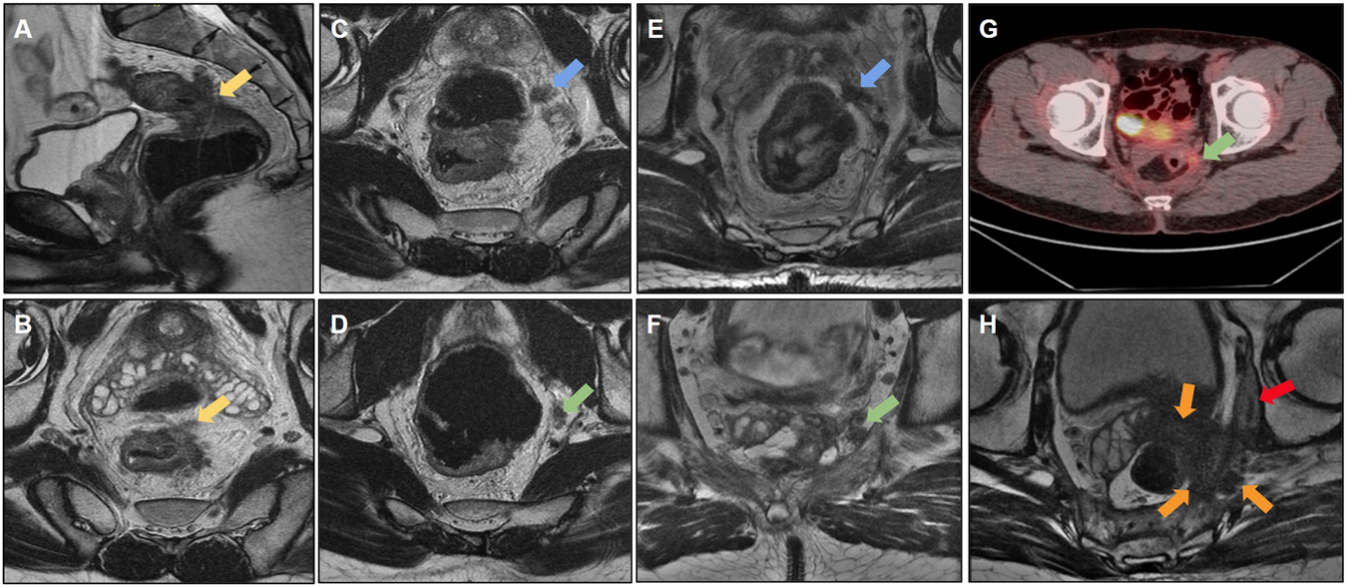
A, B Sagittal and axial T2-weighted image: baseline mrT4aN1c (yellow arrow), mesorectal fascia (MRF) positive and extramural vascular invasion positive (EMVI). C Tumor deposit in the rectoprostatic recess (blue arrow) and (D) suspicious lateral pelvic lymph node on the left (green arrow) were also characterized. E Post-long-course chemoradiotherapy (CRT) restaging demonstrates a response tumor regression grade (TRG) 3, with the persistence of the tumor deposit (blue arrow) and pelvic lateral lymph node on the left (not included in the image). F Follow-up after mesorectal excision with colorectal anastomosis revealed the presence of poorly defined tissue with intermediate signal intensity on T2-weighted imaging in the area where the previous tumor deposit and lateral pelvic lymph node were identified, raising suspicion regarding neoplastic recurrence (green arrow). G Positron emission tomography (PET)-computed tomography (CT) scan demonstrating increased radiopharmaceutical uptake in the region adjacent to the left colorectal anastomosis, corresponding to the suspicious tissue (green arrow). H At the 2-year follow-up, there was a notable progression of neoplastic tissue, characterized by infiltration of the rectum, prostate, left seminal vesicle, and pelvic lateral wall muscles (orange arrow). Notably, edema of the left internal obturator muscle is observed, likely related to denervation (red arrow)
